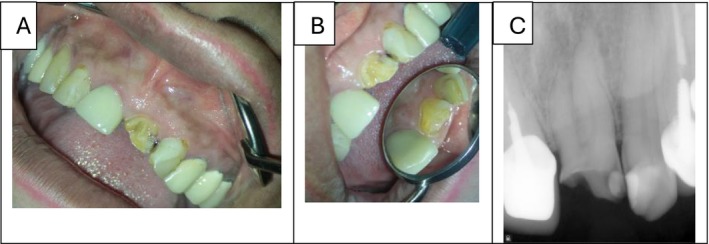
Preoperative clinical and radiographic views of the tooth #21. (A and B) Clinical photograph showing a fractured crown with a carious lesion. (C) Periapical radiograph revealing root bifurcation in the mesiodistal direction.
